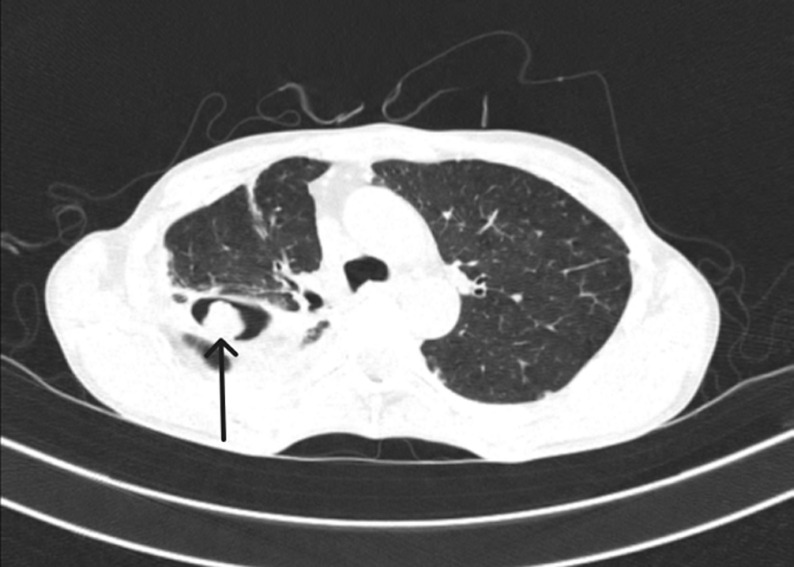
an axial section of computed tomography of lung showing a round enhancing lesion with a surrounding air shadow seen in the superior basal segment of the right lower lobe measuring 2.5 cm x 1.6 cm in size which also changed in orientation with a change in position of the patient; Monod's sign
